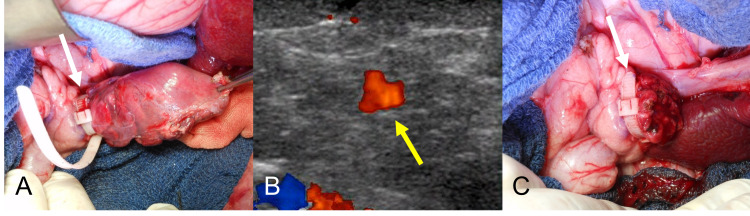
Intraoperative findings. (A) The pancreas is ligated with the pancreas ligation band. (B) Arterial blood flow to the splenic lobe is confirmed by intraoperative ultrasonography. (C) The pancreas is divided at 1 cm to the left of the ligation line. The white arrow indicates the pancreas ligation band. The yellow arrow indicates the arterial blood flow to the splenic lobe.
